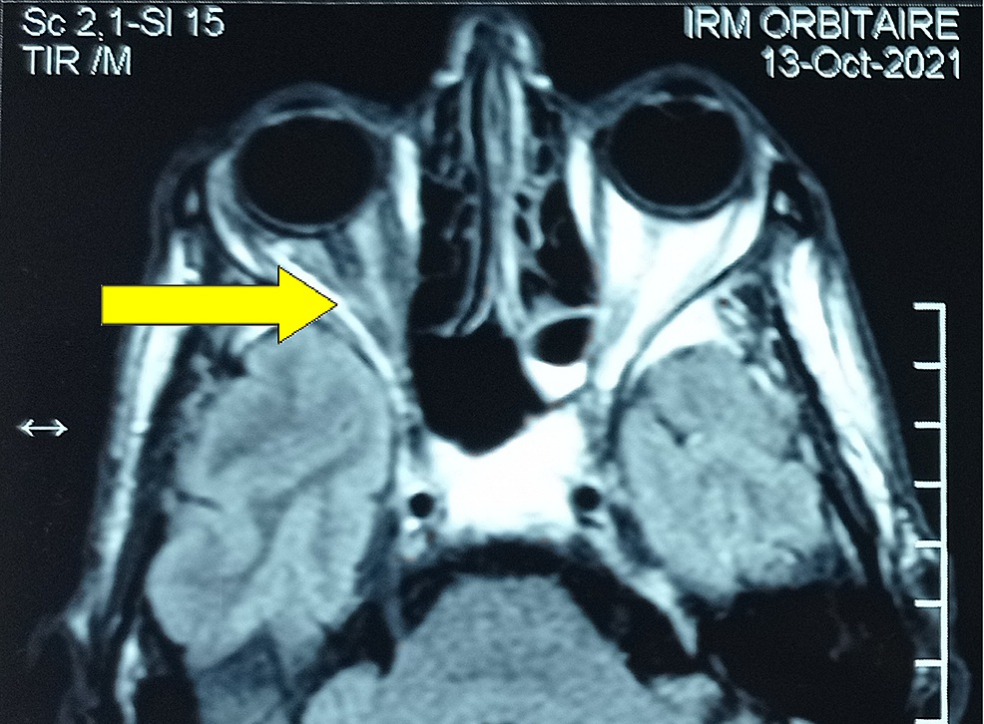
MRI imaging after six chemotherapy cycles that objectified more than 80% of response rate (yellow arrow).
